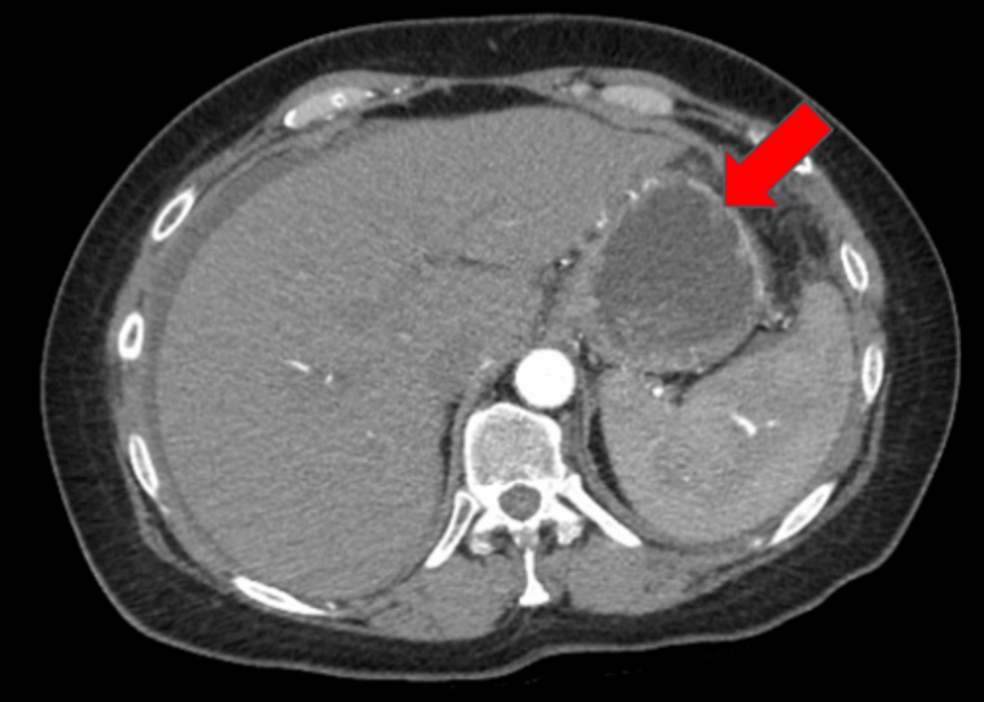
A triphasic CT scan of the liver in the venous phase, revealing increased contrast uptake of the hepatic mass suggestive of an underlying malignancy.
